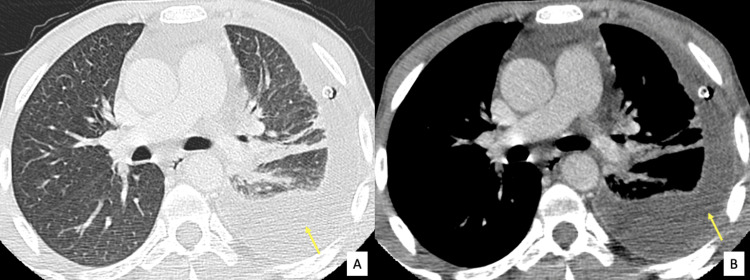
Axial Chest CT in lung parenchyma (A) and mediastinal (B) windows Left pleural effusion with passive atelectasis. No parenchymal consolidation or lung nodules were found otherwise.
